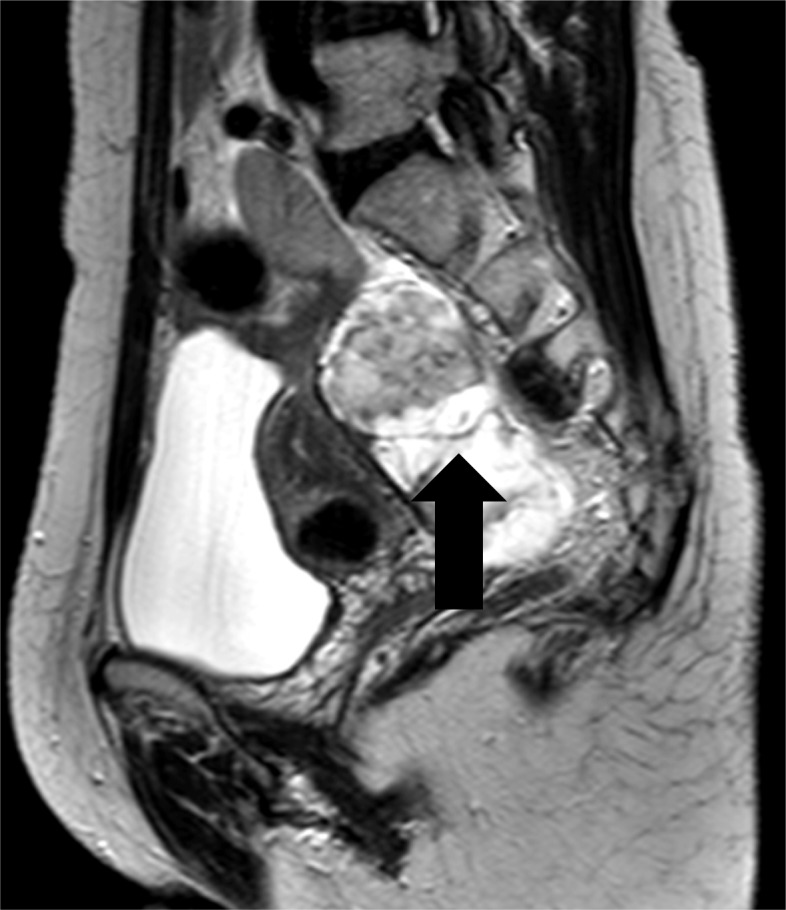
Sagittal plane of the pelvic MRI. T2-weighted MRI of the pelvis reveals a tumor with solid components on the dorsal side of the uterus. The tumor interior shows signal hyperintensity
